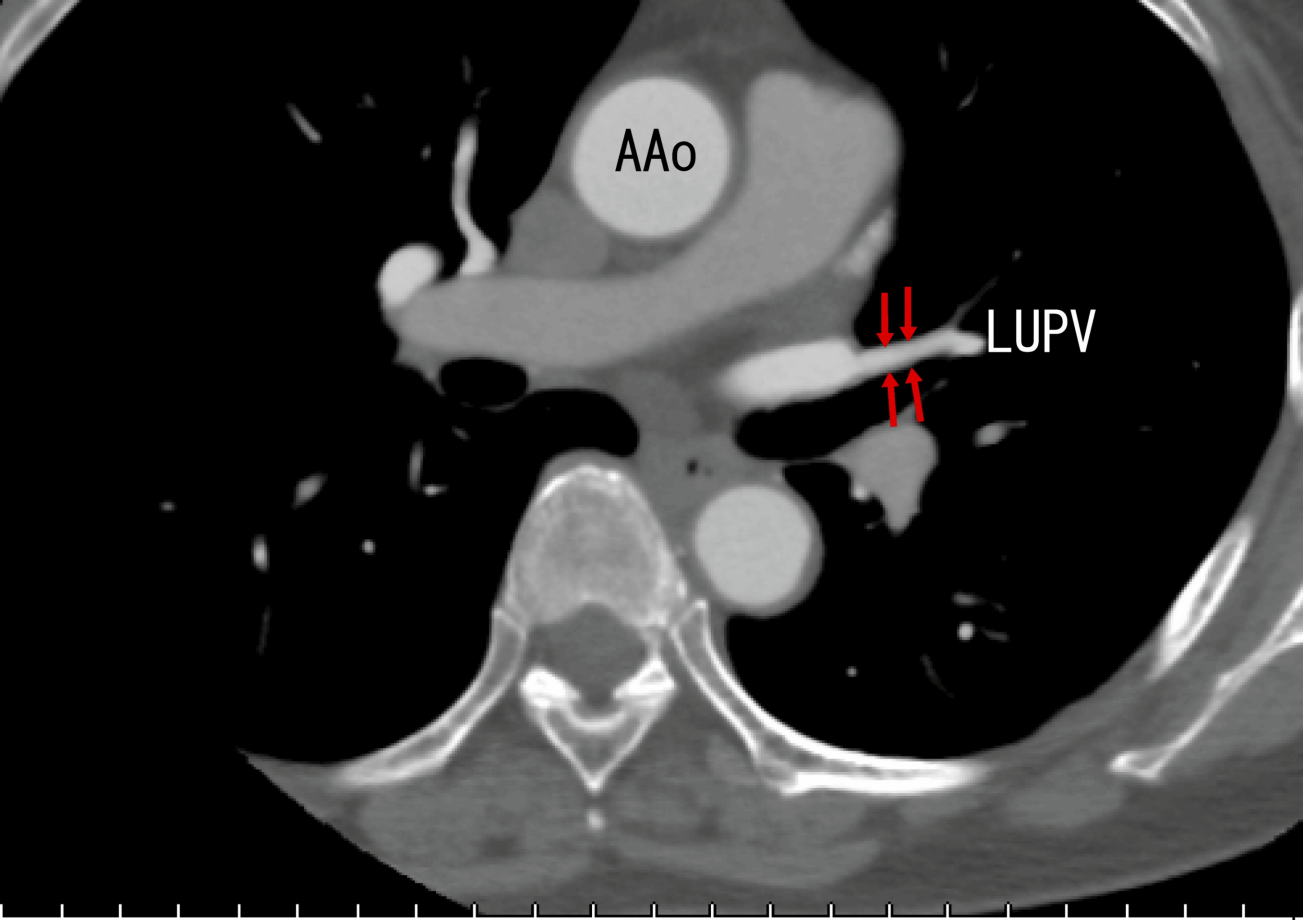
Axial-enhanced CT images Axial images depict no thrombi in the LUPV (arrows). AAo: ascending aorta, LUPV: left upper pulmonary vein
